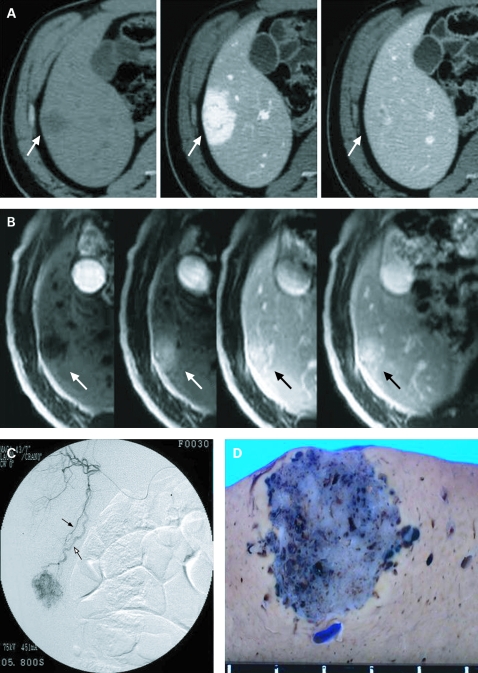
(A) CT revealed that the tumour was hypointense pre-enhancement and highly enhanced in the arterial phase with early washout (arrows). (B) Enhanced MRI after gadolinium injection. The tumour was hyperintense on T1-weighted images in the arterial phase but demonstrated prolonged enhancement in the portal venous and delayed phases (arrows). (C) Angiography showed a definite tumour with an arterioportal shunt (the artery (A5, filled arrow) and the portal vein (P5, open arrow)). (D) Grossly, the 1.5 cm soft mass in the subcapsular region showed a haemorrhagic cut surface with numerous microcystic spongiotic structures but was non-encapsulated.
